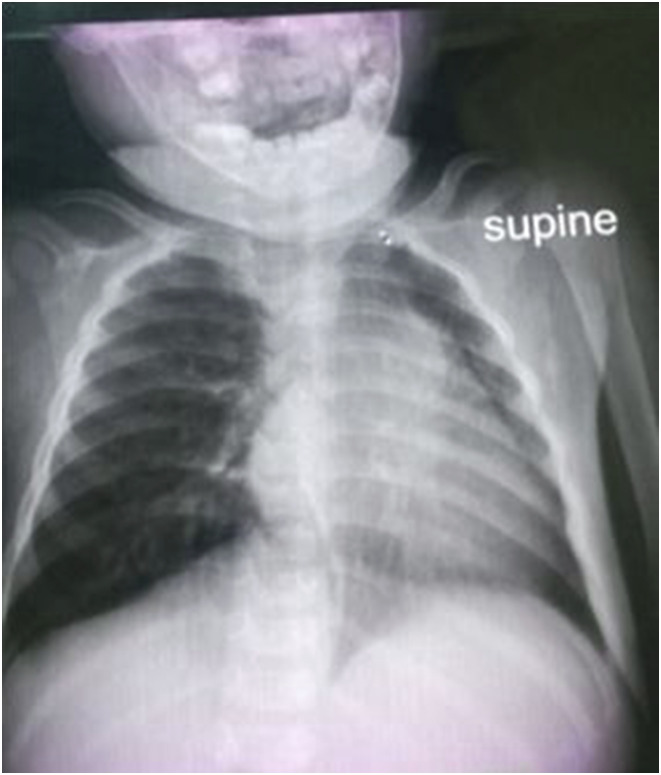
Chest X-ray of patient 2.
